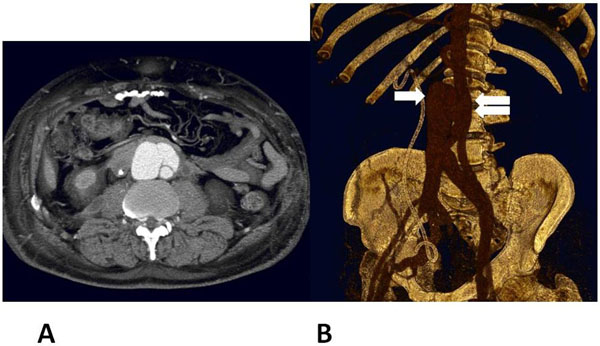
A Retroperitoneal fibrosis demonstrated at CT scan B Aorto-caval fistula originating from the abdominal aorta below the renal arteries (double arrow). The inferior vena cava (arrow) was completely occluded few centimetres above the communication with the aorta.
